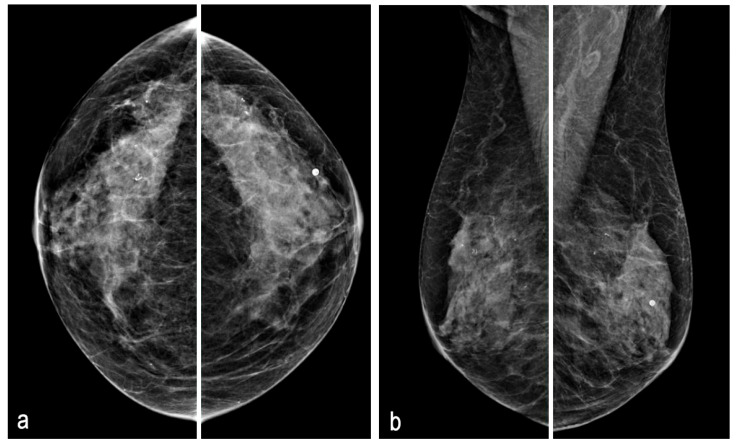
Mammographic CC (a) and MLO (b) views revealed heterogeneously dense breast, which may obscure small masses (ACR category c). However, in the UIQ, the nodular uptake reported on the PET-CT was identified as a 10 mm mass with an irregular shape and partially obscured margin. No prior mammographic studies were available for comparative purposes. No suspicious microcalcifications were observed. In other studies, metastases have been reported on mammography as well circumscribed, round, or oval masses without calcifications. Less commonly, they may present as spiculated masses [14,15].
